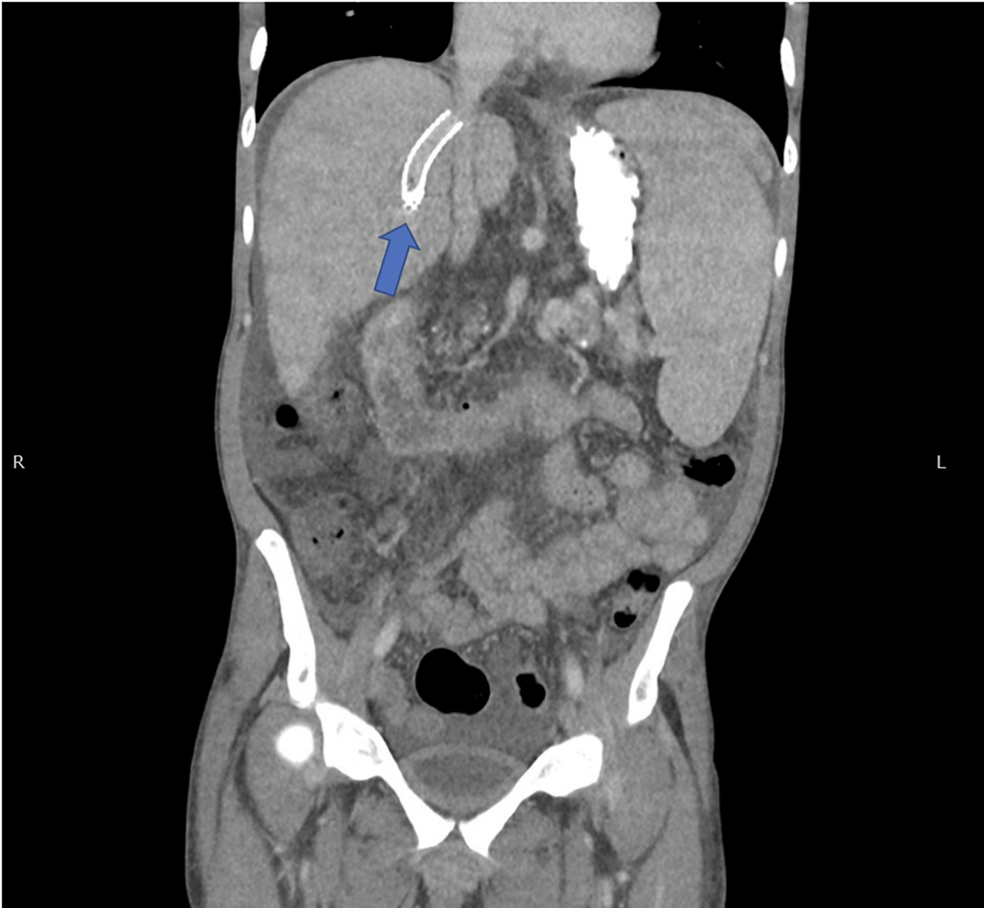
Computed tomography imaging demonstrating patency of the transjugular intrahepatic portosystemic shunt catheter.
